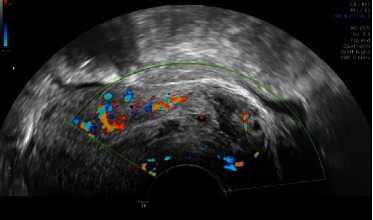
Color Doppler study did not provide new data for diagnosis.
